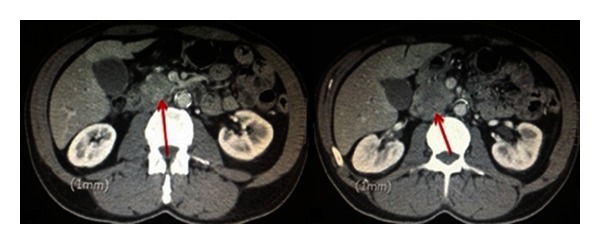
CT abdomen revealing a bulky pancreatic head.
